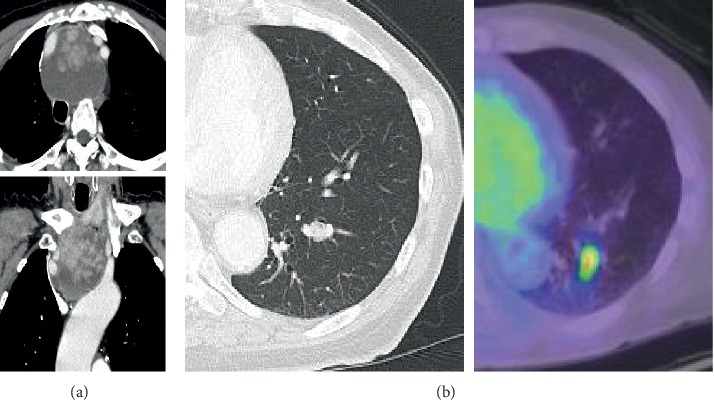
CT images. (a) 7.2 cm mass in the anterior mediastinum. (b) Pulmonary metastasis.
